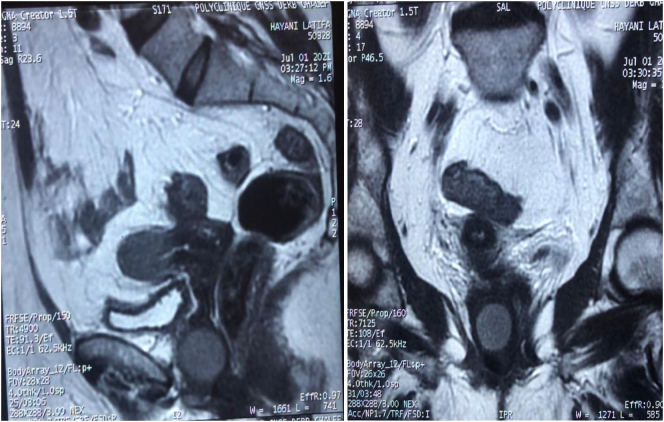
MRI appearance of the intra-vaginal mass.
